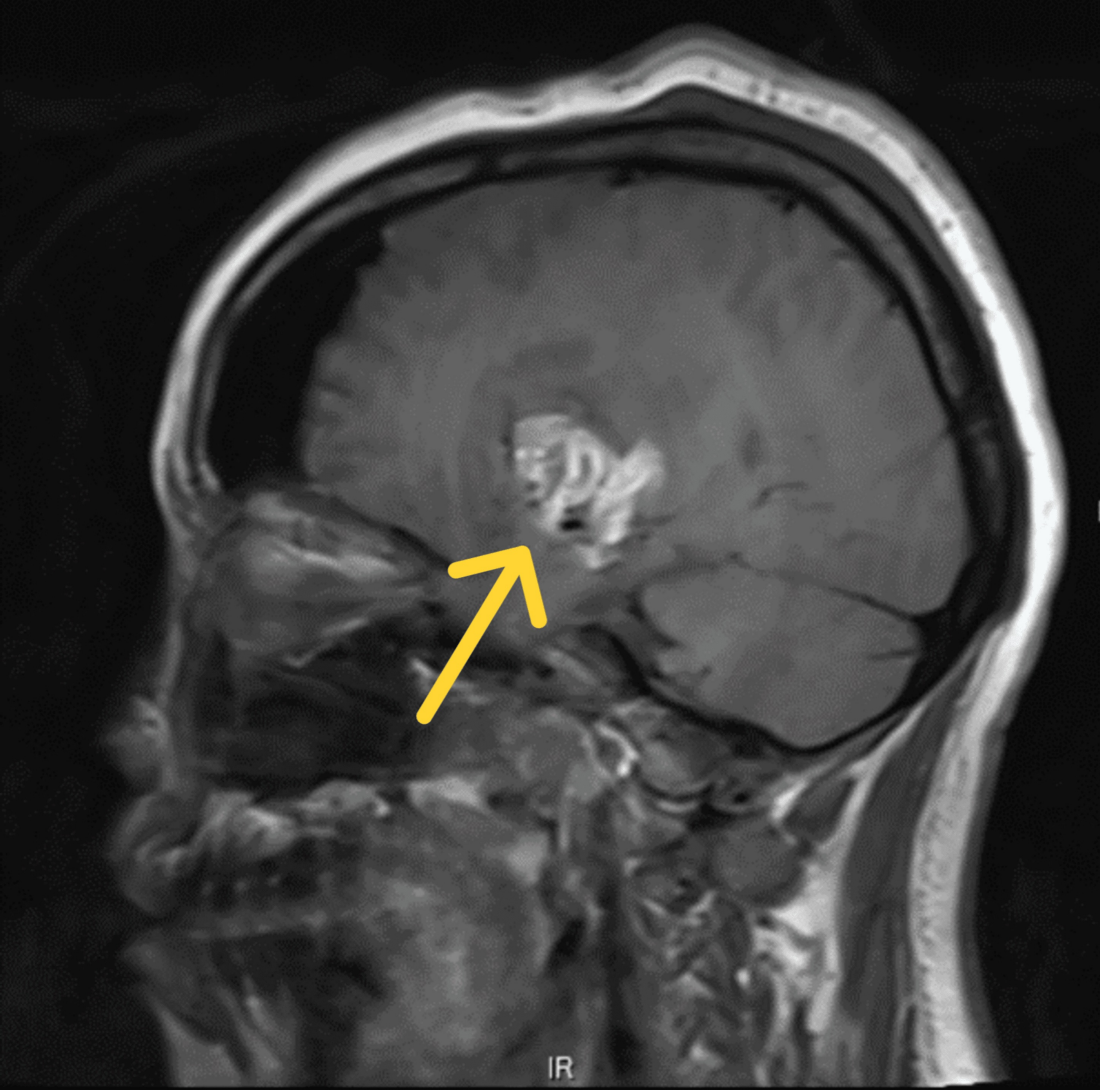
Postoperative MRI after second surgery (T1 with contrast, sagittal view).
